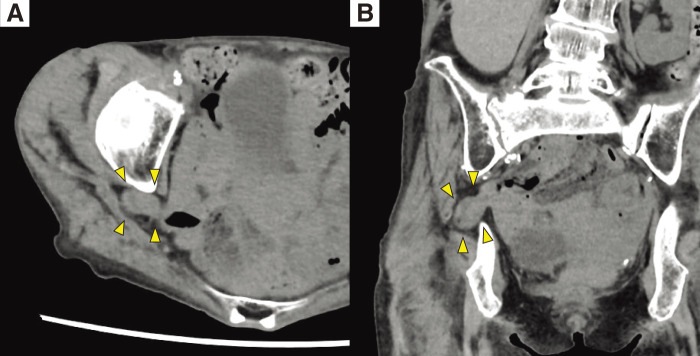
CT findings. (A) Axial view. (B) Coronal view. Abdominal CT revealed the small-bowel herniated through the sciatic foramen (arrowheads), with no evidence of bowel obstruction or ischemia.
